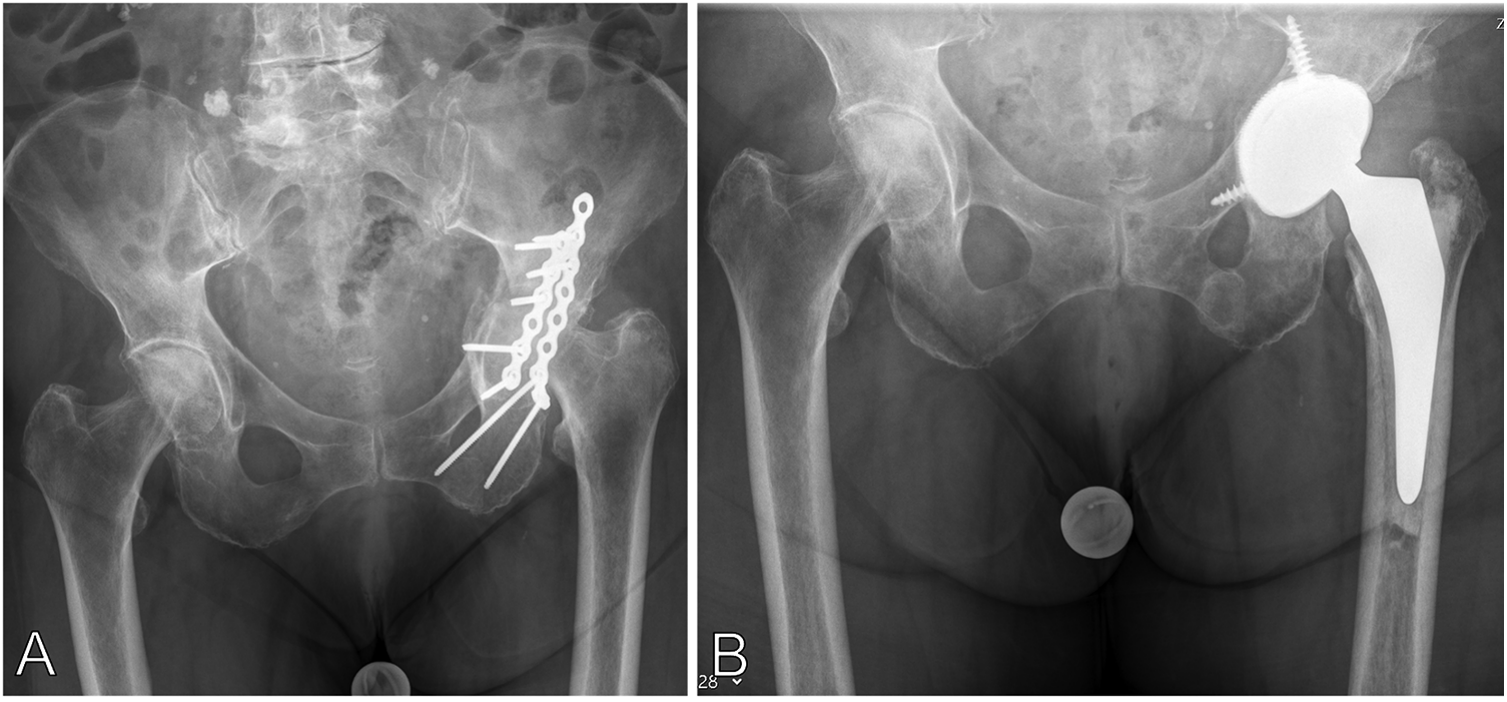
(A) AP pelvis x-ray showing partial femoral head necrosis and acetabular protrusion after open reduction and internal plate fixation of a posterior column acetabular fracture via a Kocher-Langenbeck approach. (B) Postoperative anterior posterior (AP) Pelvis 6 weeks after implantation of a hybrid cemented total hip arthroplasty using a cementless revision cup with additional screw fixation and a cemented stem.
